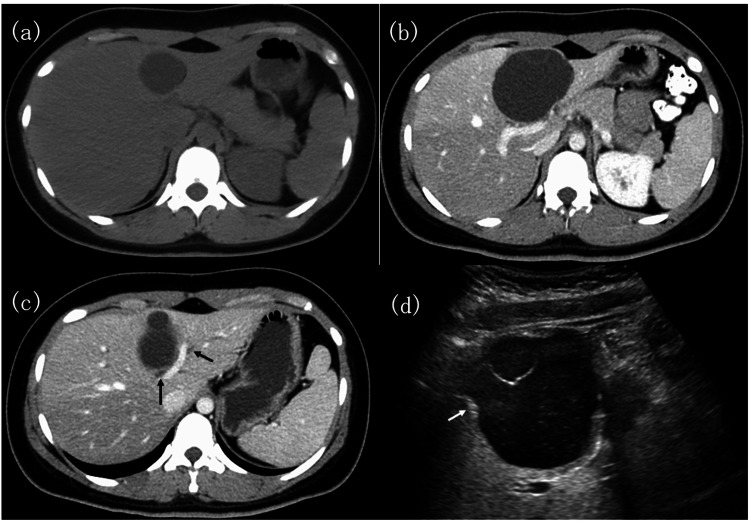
Abdominal computed tomography and abdominal ultrasonography findings a: Computed tomography (CT) showing a cystic lesion in the medial section of the liver measuring 35 mm. b: Contrast-enhanced CT showing a multi-ocular cystic tumor in the medial section of the liver with calcification in the cyst wall. The tumor measured 75 mm in diameter after 8 months. c: Dilation of the left hepatic duct and anterior segment bile duct (black arrow). d: Ultrasonography showing a multilocular cyst and a hyperechoic nodule of 6 mm in size (white arrow).
